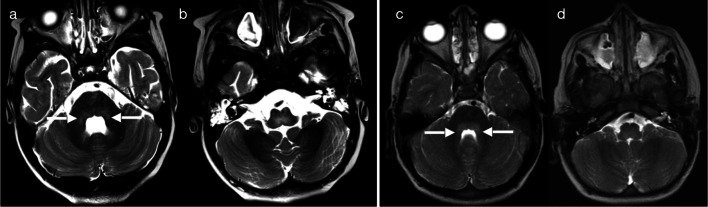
T2 hyperintensity in posterior fossa—dorsal pons (Pattern 6A). Case 1: 4-year-old child with fluctuating GCS. Stool was positive for enterovirus (serotype E-71). T2-weighted imaging (a, b) demonstrated hyperintensity of the dorsal pons and medulla, with extension of signal abnormality into the middle cerebellar peduncles bilaterally (arrows). Case 2: 3-year-old child with lethargy, vomiting and fever, as well as right facial nerve palsy and unsteadiness on feet. Stool was positive for enterovirus (serotype not known). T2-weighted imaging (c, d) demonstrated hyperintensity of the dorsal pons and medulla, with extension of signal abnormality into the middle cerebellar peduncles bilaterally (arrows)
